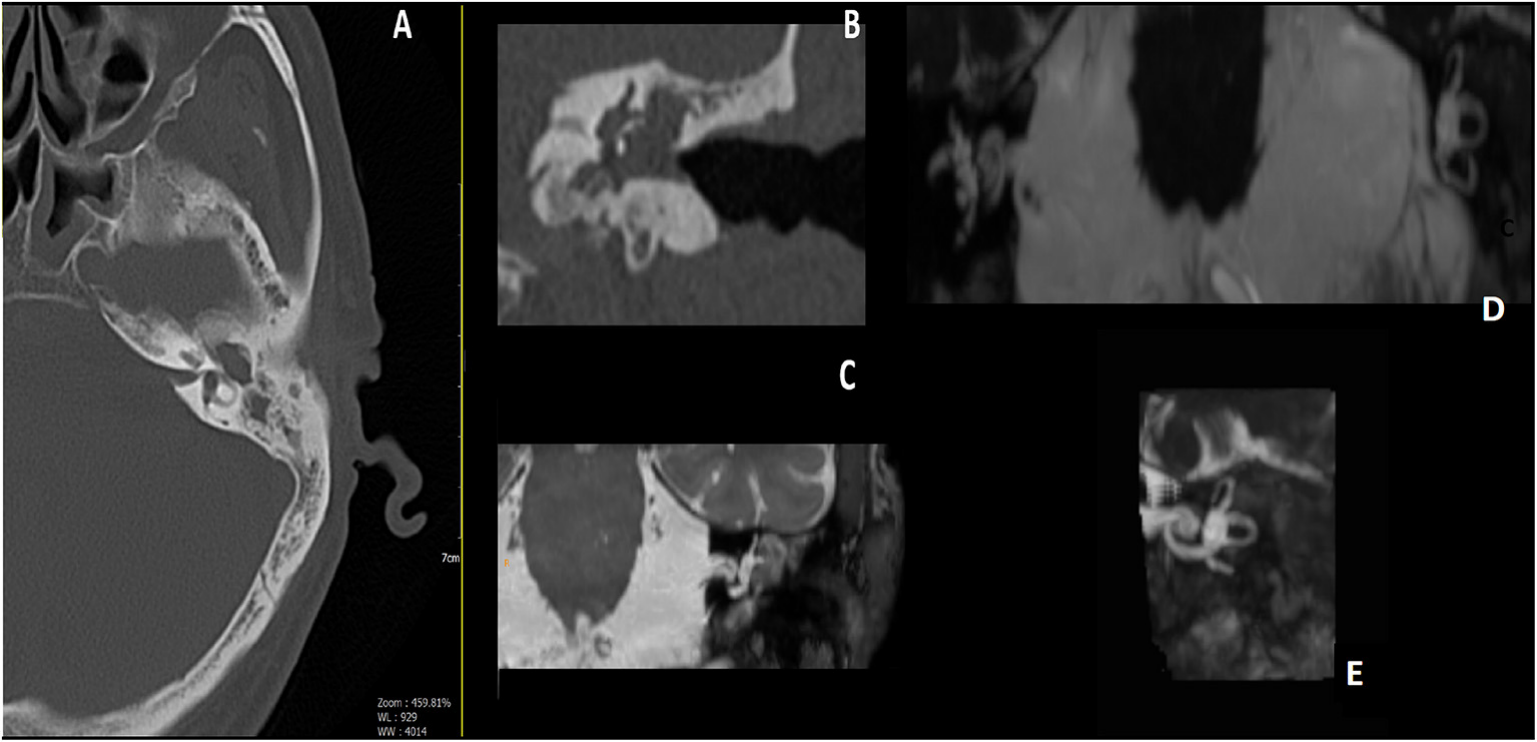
Male, 65 years old; (A) pre-operative axial CT scan, erosion of the bony lateral semicircular canal (LSC); (B) pre-operative Coronal CT scan, a well-evident erosion of the LSC; (C) pre-operative MRI, tissue appears to encase the LSC. (D) Post-operative MRI 3D Fiesta 3D sequences (field of view 20 × 20 cm TR 4 ms; TE 2 ms; flip angle 55°); (E) post-operative images Maximum Intensity Projection (MIP) for cochlea and semicircular canal visualization, detail of operated side, showing correct visualization of endocanal fluid preservation, and meaning preservation of hearing.
